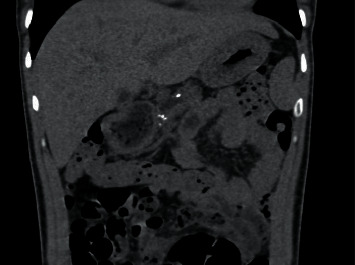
Coronal noncontrast CT through the upper abdomen shows calcifications along the body of the pancreas and mild dilatation of the pancreatic duct measuring up to 9.7 mm.
